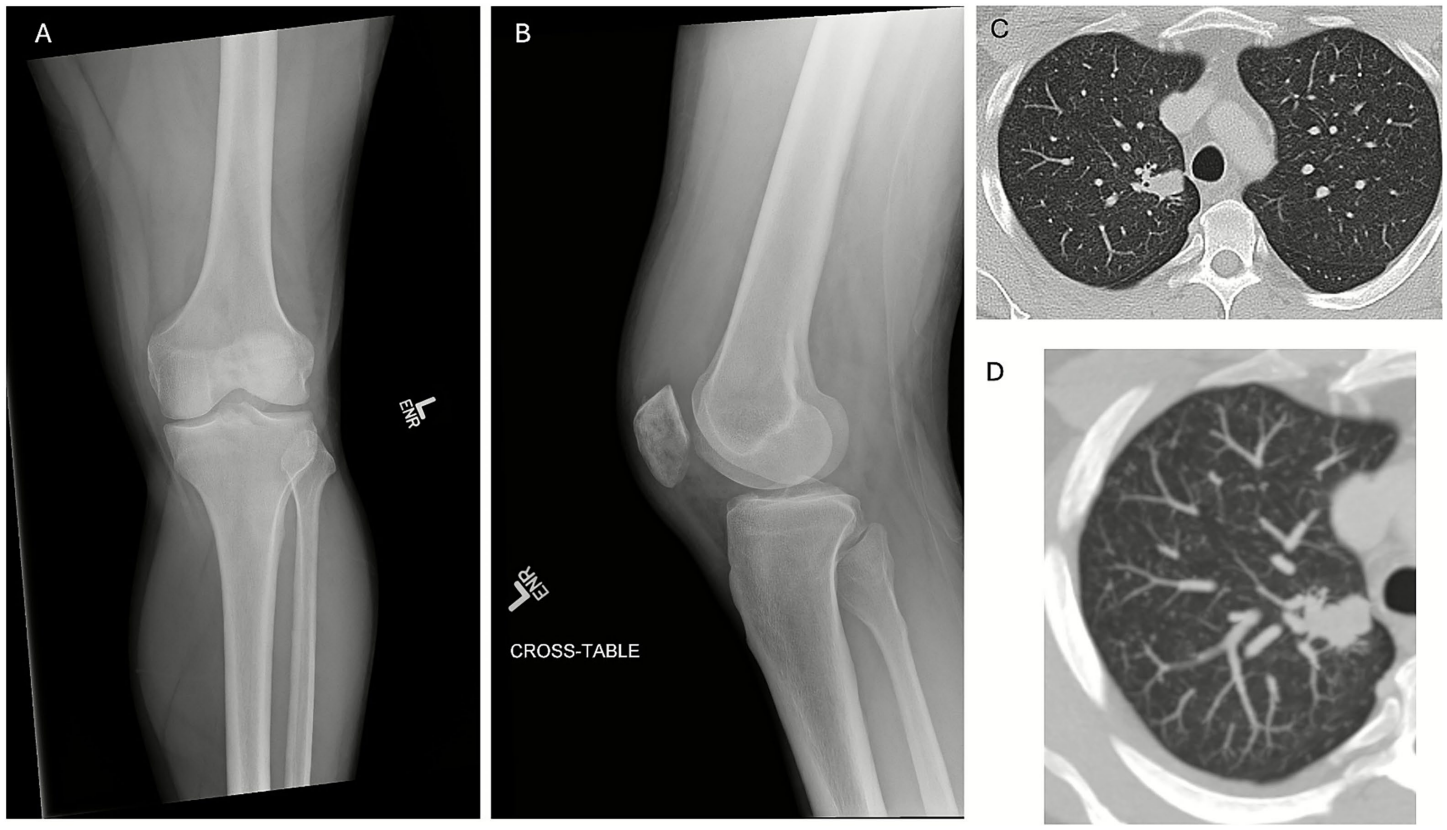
Radiographic and imaging findings of disseminated blastomycosis. (A) Anteroposterior and (B) lateral radiographs of the left knee demonstrate joint effusion with preserved joint spaces and patchy lucency in the patella. (C) Chest computed tomography showed focal consolidation in the apical segment of the right upper lobe and diffuse bilateral non-calcified miliary micronodules. (D) Maximum intensity projection (MIP) CT image better demonstrating diffuse micronodularity.
